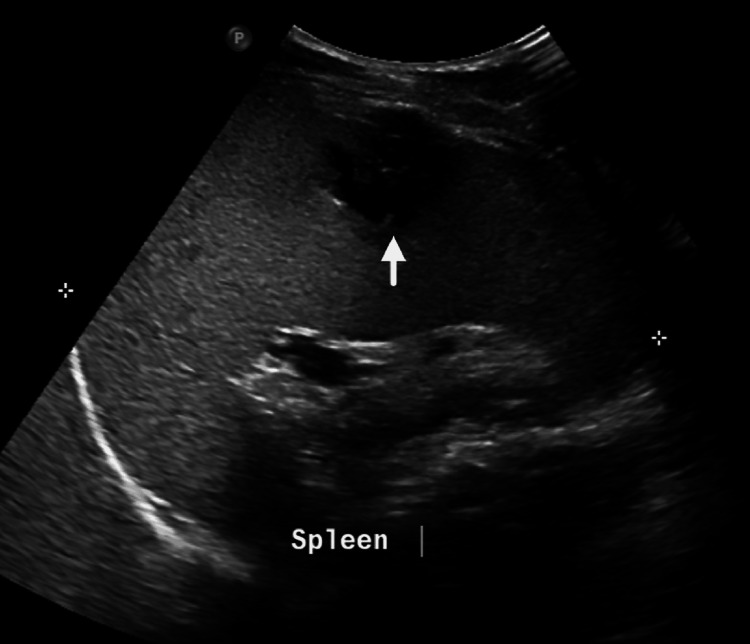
USG abdomen showing mild splenomegaly with a solitary heterogenous hypoechoic lesion suggestive of an abscess USG: ultrasonography.
